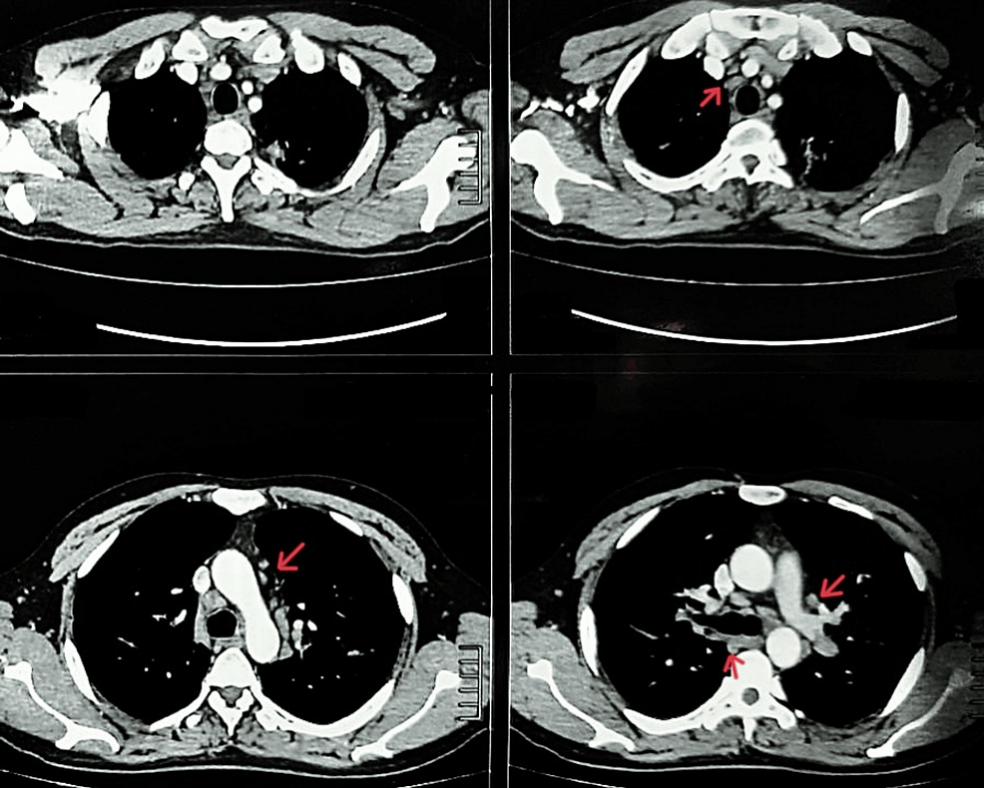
Computed tomography of the chest showing enlarged bilateral hilar lymph nodes.
